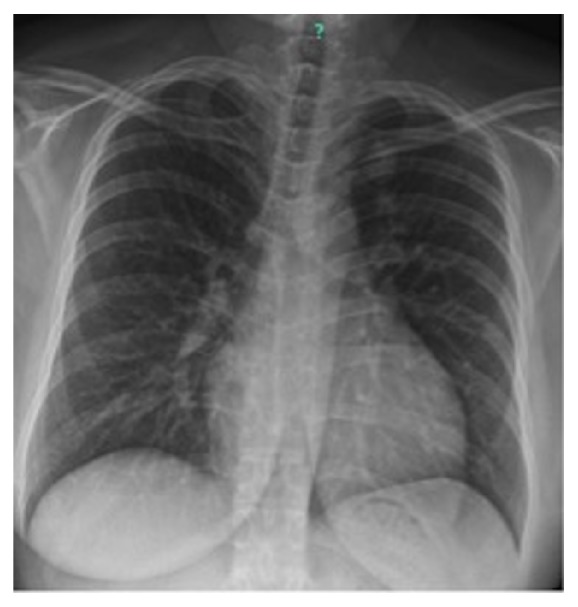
Chest X-Ray showing 2 left upper lobe lung masses.
